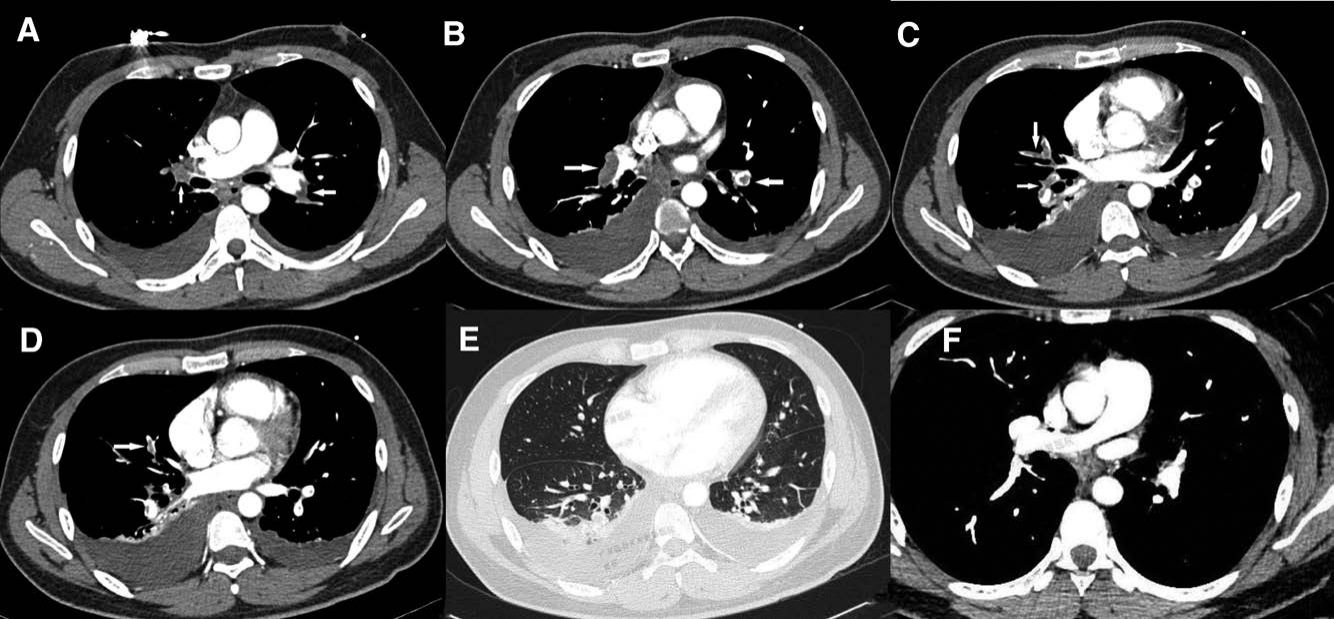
Lung CT during hospitalization and CTPA after discharge (arrow indicates pulmonary filling defect). CT = computed tomography, CTPA = computed tomography pulmonary angiography.
